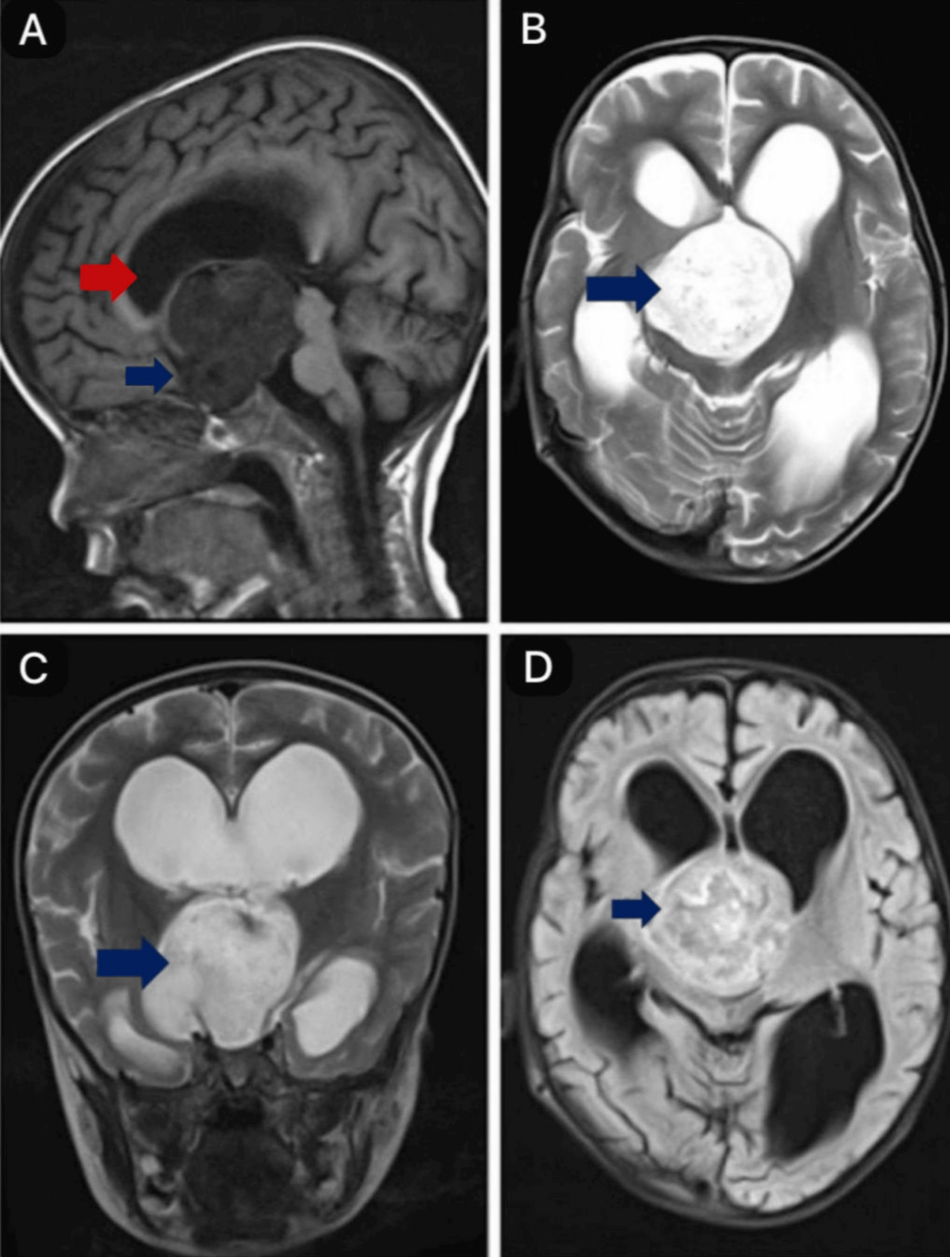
MRI brain A) A T1-weighted sagittal section image shows a heterogeneously hypointense tumor causing effacement of the 3rd ventricle (indicated by a blue arrow), causing proximal obstructive dilatation of the lateral ventricle (indicated by a red arrow). B and C) T2-weighted axial and coronal sections show heterogeneously hyperintense tumors in the suprasellar region (indicated by a blue arrow). D) FLAIR image showing a heterogeneously hyperintense tumor with central/cystic areas showing suppression (indicated by a blue arrow). MRI: magnetic resonance imaging; FLAIR: fluid-attenuated inversion recovery
